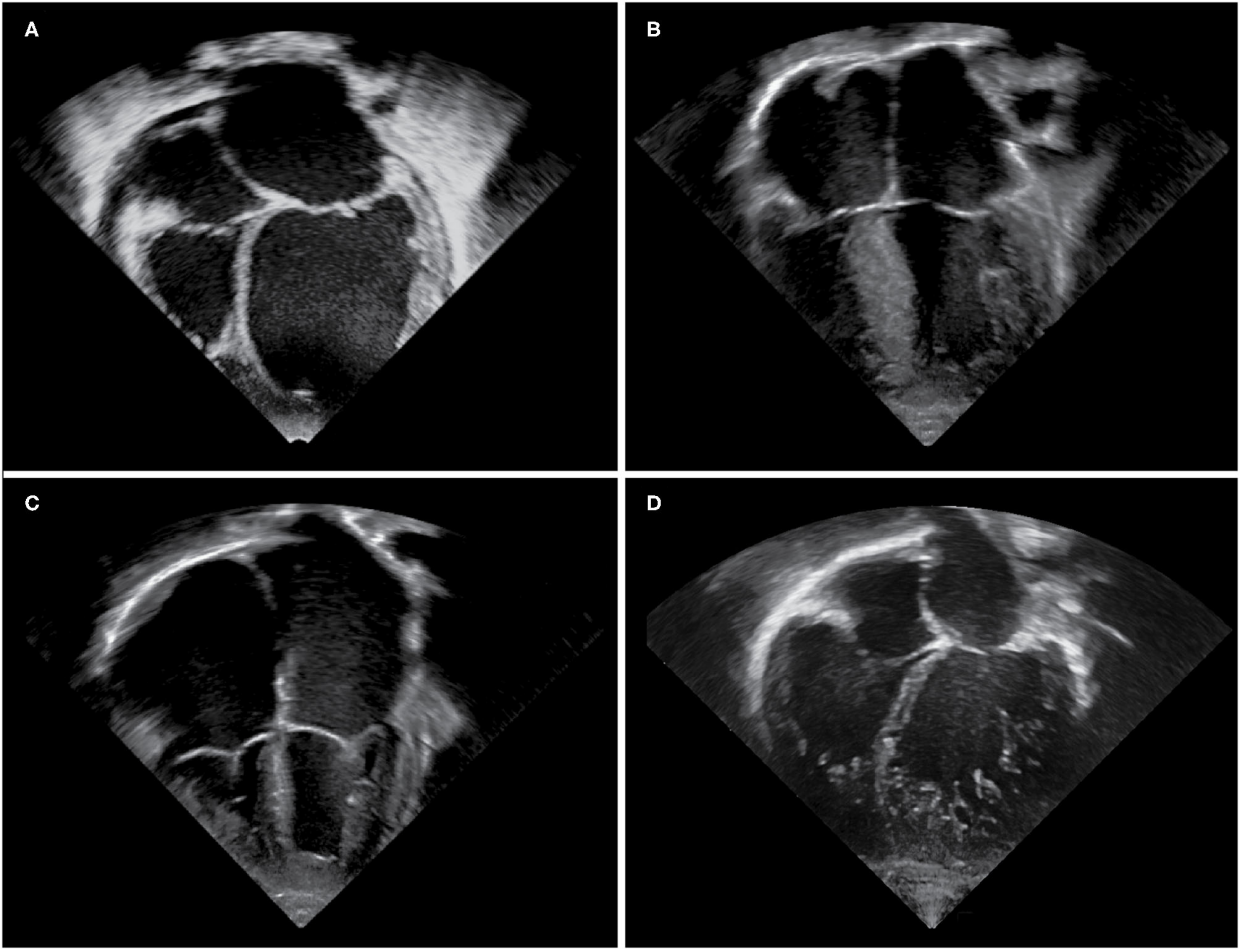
Echocardiographic images of cardiomyopathy phenotypes. Apical four chamber views demonstrating (A) a dilated left ventricle and left atrium in a DCM patient, (B) hypertrophy of the interventricular septum and left ventricular free wall in a HCM patient, (C) massively dilated atria and small right and left ventricular cavities in an RCM patient, (D) an extensively trabeculated myocardium with a compacted and non-compacted layer and deep intertrabecular recesses most prominent at the left ventricular apex and free wall in an LVNC patient.
